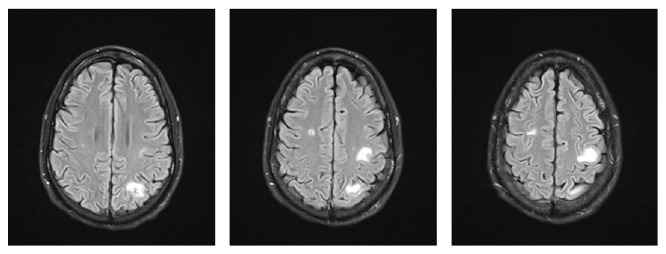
MRI of the brain revealing new interval hemorrhagic and nonhemorrhagic metastases within the bilateral parietal lobes and left occipital lobe visible on T2 FLAIR. This was found after starting chemotherapy with 4 cycles of bleomycin, etoposide, and cisplatin.
